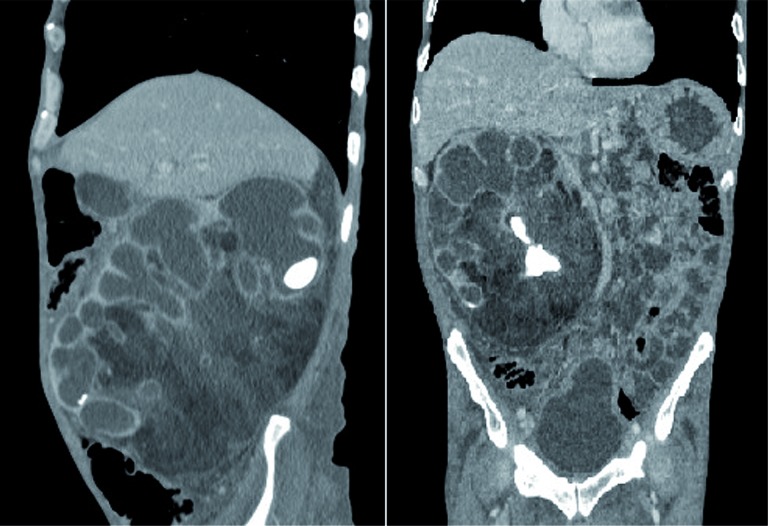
Sagittal and coronal CT images show calculi, dilated calyces and renal parenchymal atrophy and exuberant fibrofatty proliferation.
